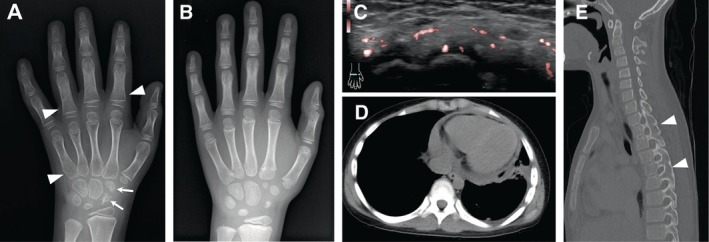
Two cases with late consequences of systemic JIA. (A–C) A 6‐year‐old boy with a long‐standing history of systemic JIA since 2 years of age. Frontal radiograph of the left hand (A) showed dysplastic carpal bones (arrows), mild metaphyseal widening of short tubular bones (arrowheads), and juxta‐articular osteopenia, compared with an age‐matched control (B). US (C) showed synovial thickening with increased power Doppler signal along tendon sheaths, suggesting active tenosynovitis/synovitis. (D, E) An 11‐year‐old boy with relapsed systemic JIA since 2 years of age. Non‐contrast‐enhanced CT showed pericardial and pleural effusions (D), and ankylosis of the posterior elements in the cervicothoracic spine (arrowheads) due to chronic inflammatory arthritis of the facet joints (E).
